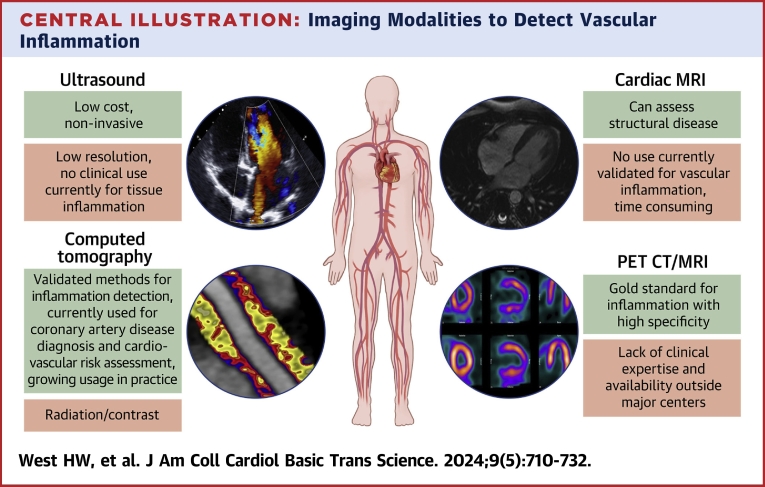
Imaging Modalities to Detect Vascular Inflammation Summary of the clinical imaging modalities in use or under development for the noninvasive detection of vascular inflammation. CT = computed tomography; MRI = magnetic resonance imaging; PET = positron emission tomography.
